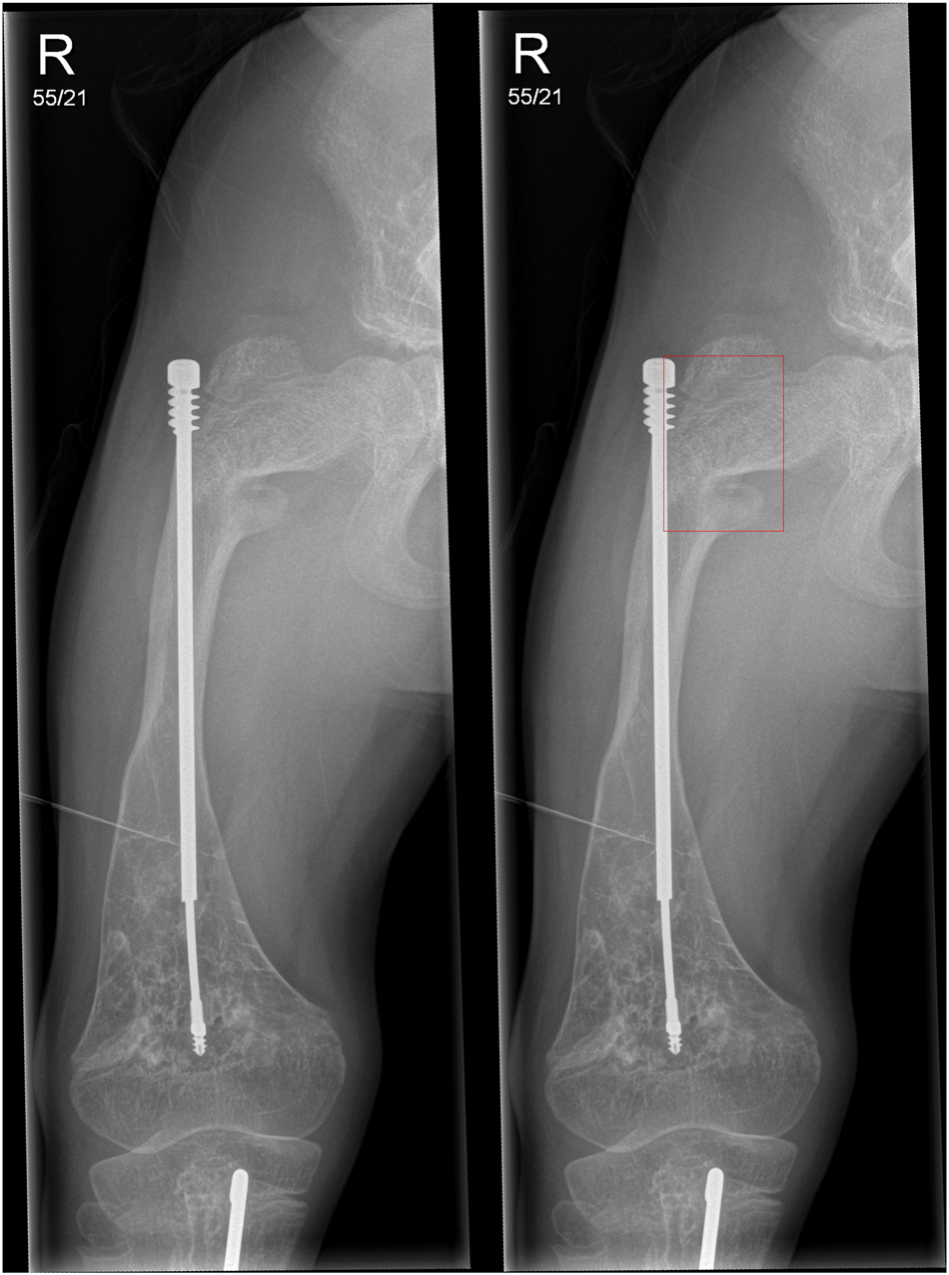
Frontal projection of the right femur with an intramedullary rod, without any acute or healing fractures, in a male patient aged 12 years old. In this example, none of the radiologists detected a fracture on the radiograph; however, the AI produced a false positive detection for a fracture (red box) at the physis of the greater trochanter. Radiologists were confident in their decision and did not change their answers from the first round to the second round. Left: Unmarked radiograph. Right: Radiograph with AI detection indicated by red box
